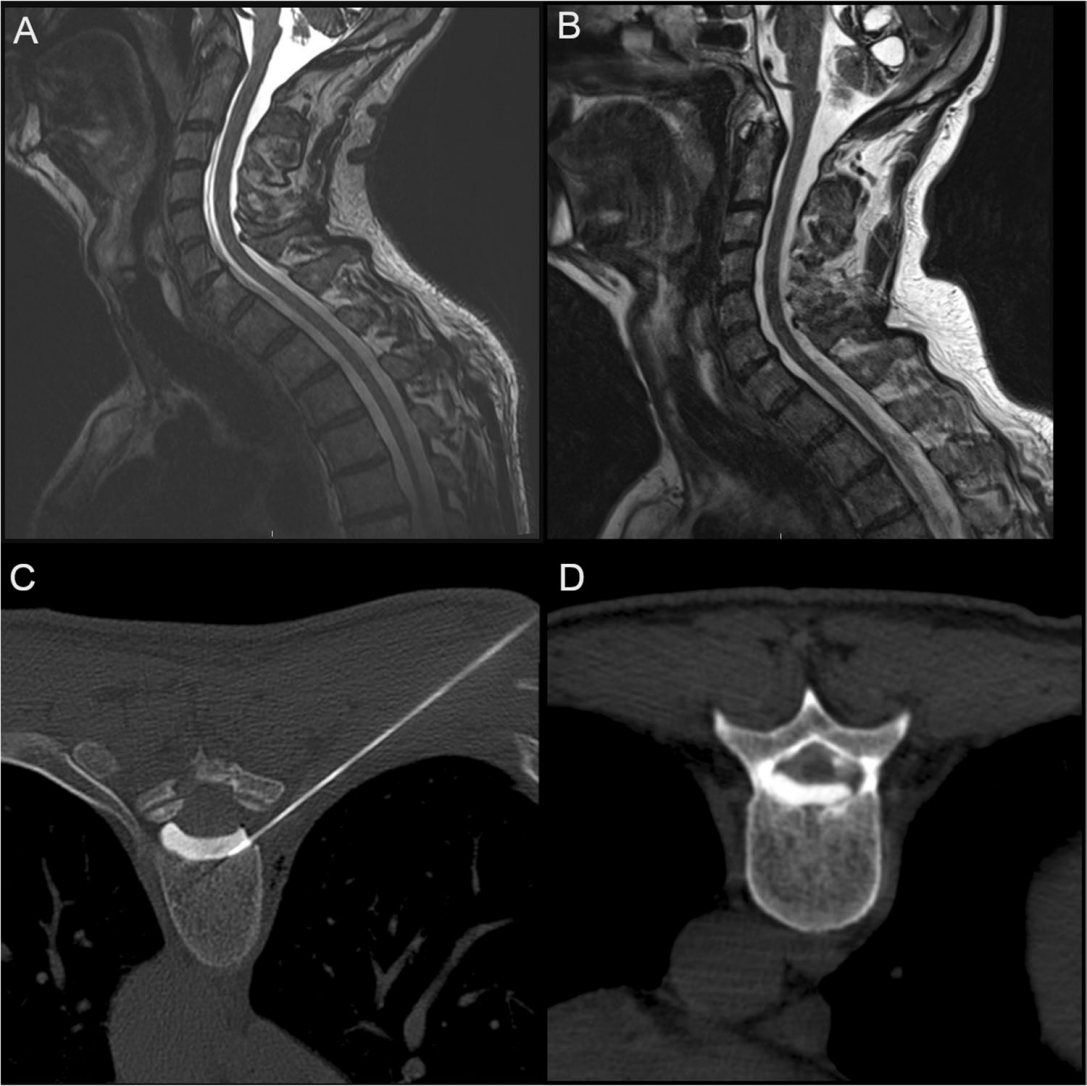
Sagittal MRI spine T2WI SPC revealing a large ventral epidural fluid collection extending from C3 to L3 (A) and post-operative resolution (B). Reverse dynamic CT myelogram demonstrating contrast injection into the ventral epidural collection (C) and subsequent tracking of contrast to the right posterior thoracic cord delineating the site of the dural defect, T9-10 (D)
